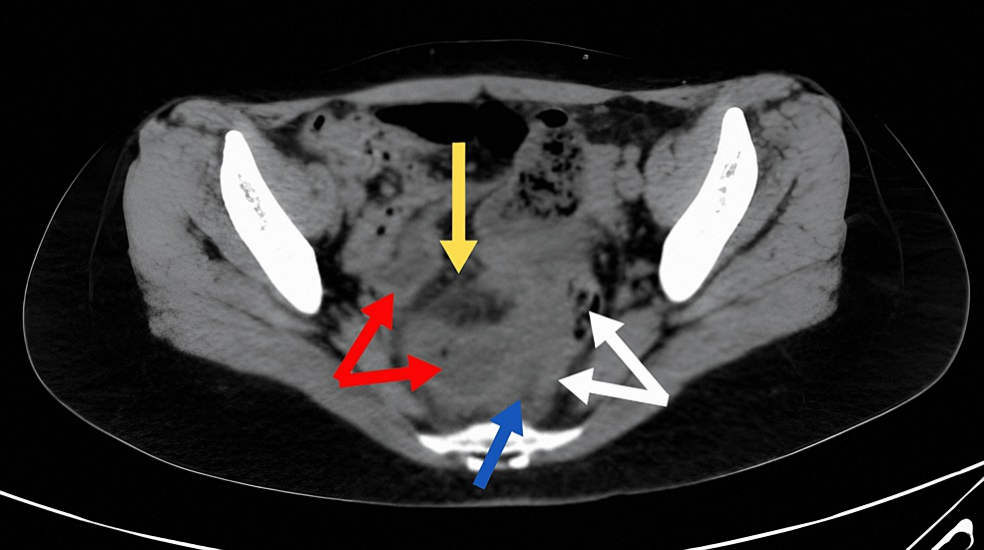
Abdominopelvic CT scan (axial view) shows the presence of dilated small bowel loops with thick wall (red arrows) in proximity to the sigmoid colon (white arrows) surrounded by pelvic free fluid (blue arrow) and dirty mesenteric fat (yellow arrow).
